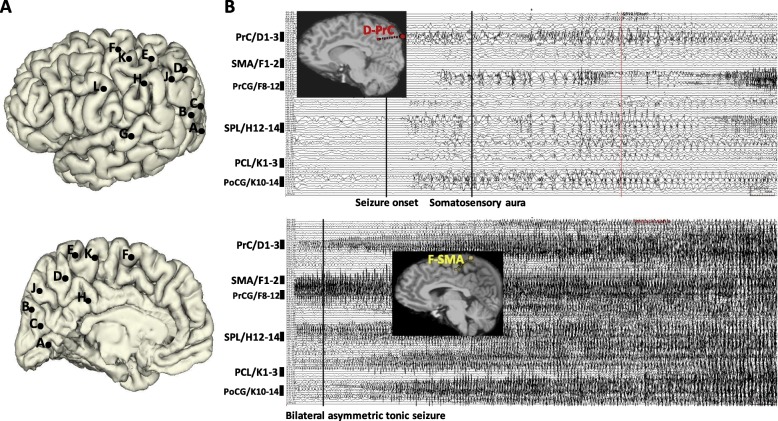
a SEEG electrode implantation on lateral and medial view 3D brain of Patient 9. b Ictal SEEG trace and 2D T1-weighted sagittal images with 3D electrodes showing the key target points. It shows that the seizure onset was recorded from PrC (medial contacts of electrode D1–3) with no clinical sign being observed. When the seizure spreads to SMA (medial contacts of electrode F1–2), PrCG (lateral contacts of electrode F8–12), PCL (medial contacts of electrode K1–3), and PoCG (lateral contacts of electrode K10–14), the semiology of bilateral asymmetry tonic seizure appeared
